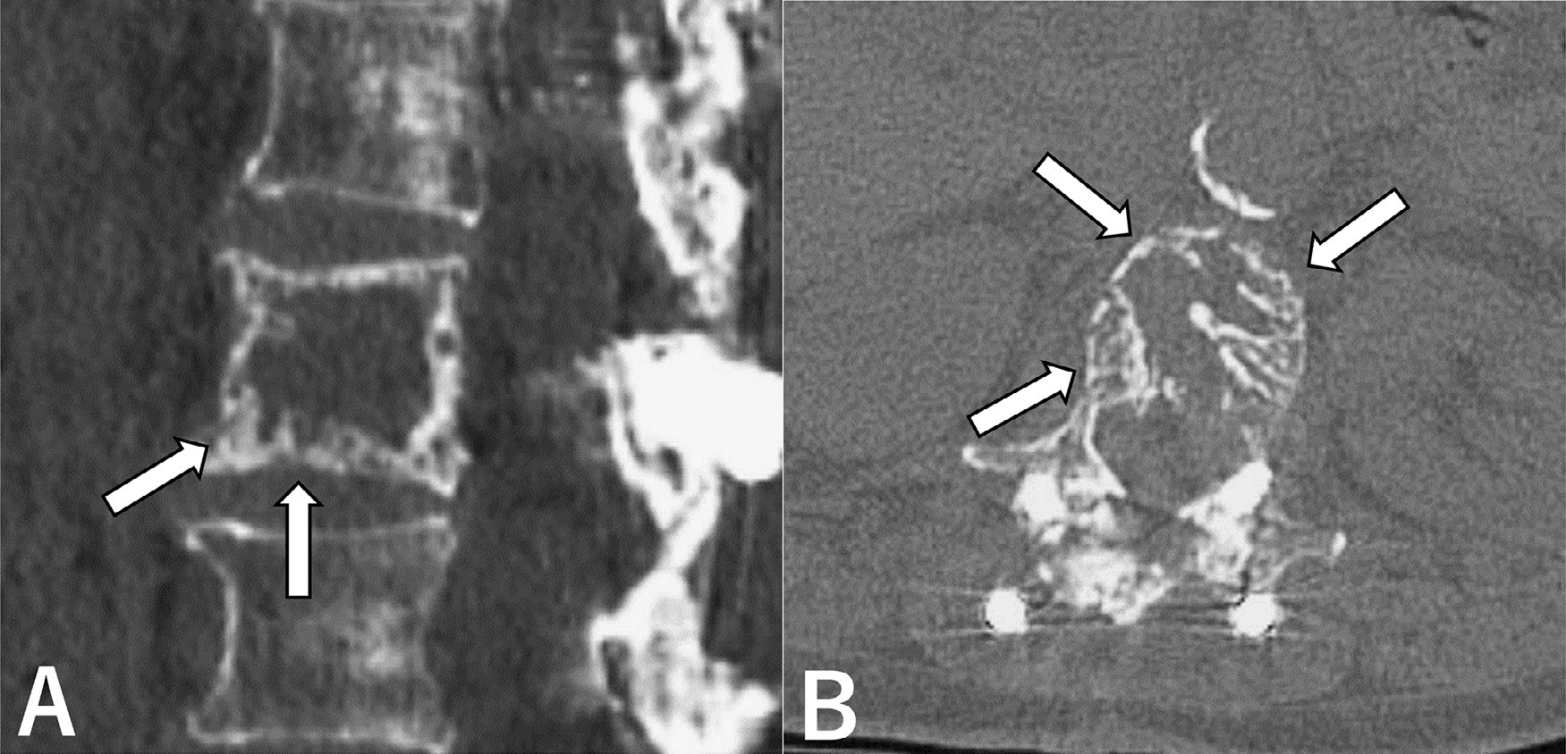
One-year follow-up CT scans. (A, B) The texture of the vertebral centrum partially regains its normal appearance (arrows). CT, computed tomography.
